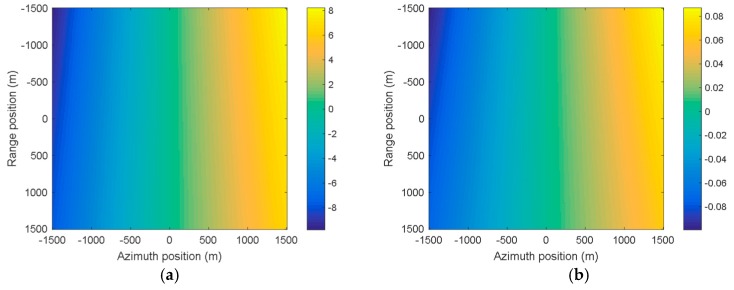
The spatial variance of the Doppler parameters. (a) The Doppler rate; (b) the derivative of the Doppler rate.
